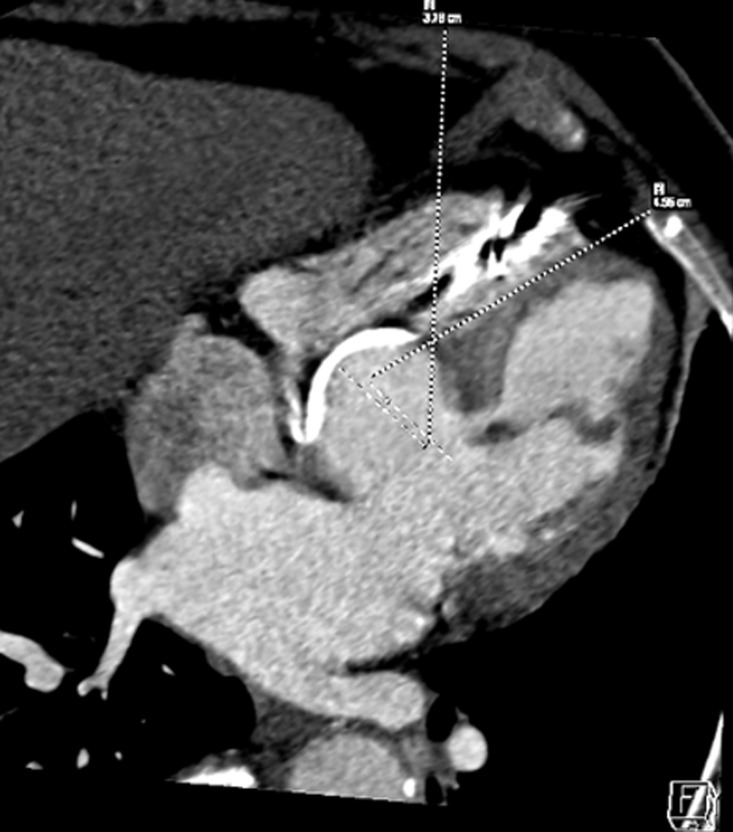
cardiac CT scan showing a heavily calcified aneurysm
